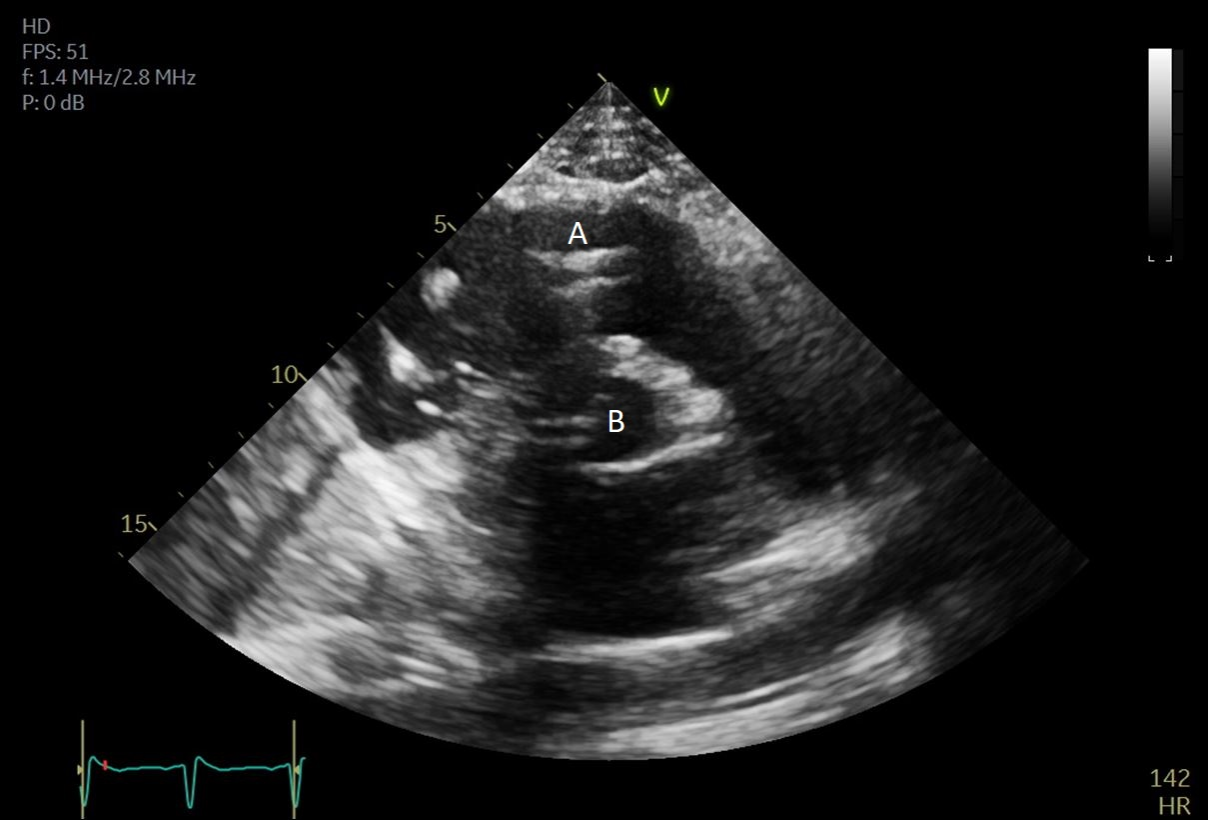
A parasternal short axis view at the level of the aortic valve
